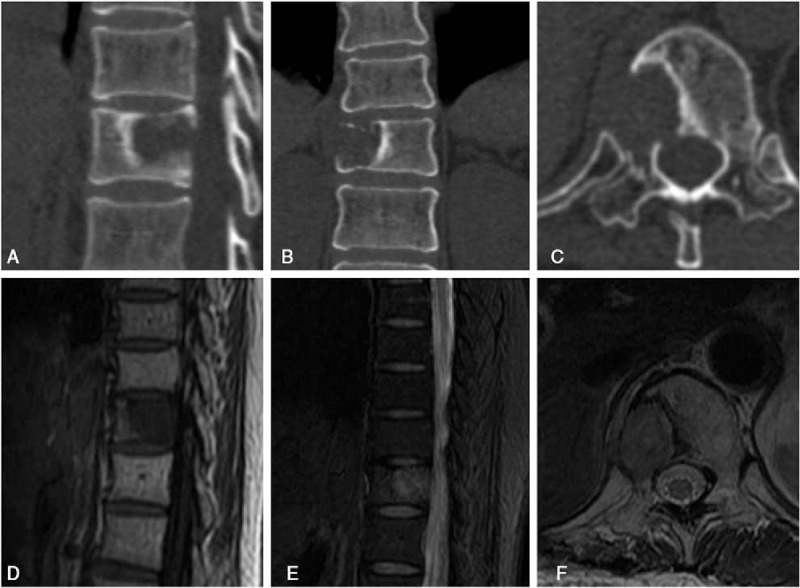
Preoperative 3-dimensional computed tomography (CT) scan image and magnetic resonance imaging (MRI). (A) Sagittal CT; (B) coronal CT; (C) axial CT; (D) T1 sagittal MRI; (E) T2 sagittal MRI; (F) axial MRI.
